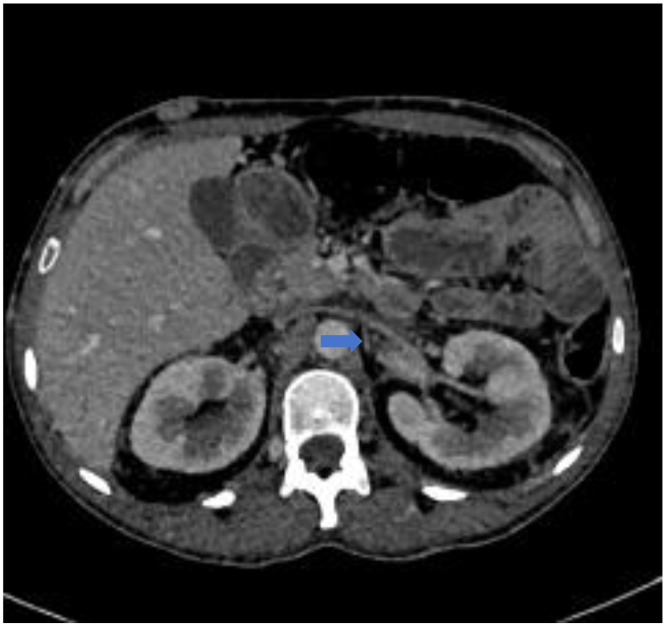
Abdomino-pelvic CT scan: Axial plan through the renal veins showing transaortic renal drainage (blue arrow).
